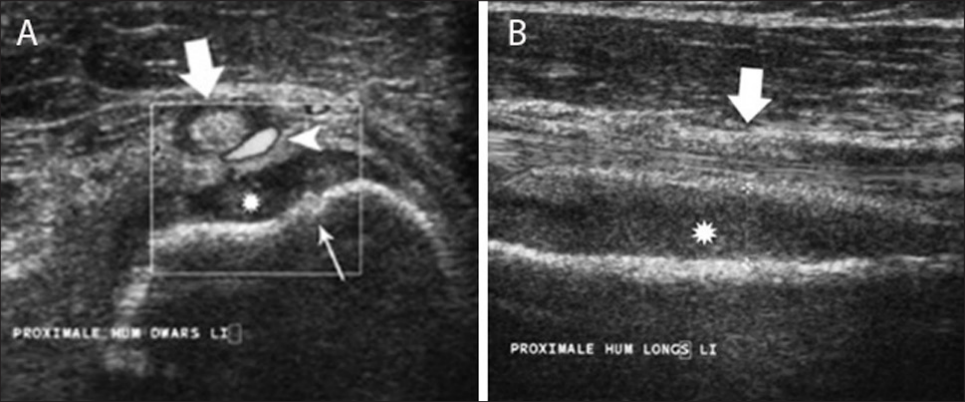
Acute childhood osteomyelitis of the proximal humerus on ultrasound. Transverse (a) and longitudinal (b) ultrasound images of the proximal humerus. Note focal thinning of the humeral cortex (thin white arrow) on the axial images in keeping with a cortical penetration of the infection causing subperiosteal pus collection (asterisk). There is also increased Doppler signal (white arrow head) within the synovium of the long head of the biceps tendon (large white arrow).
